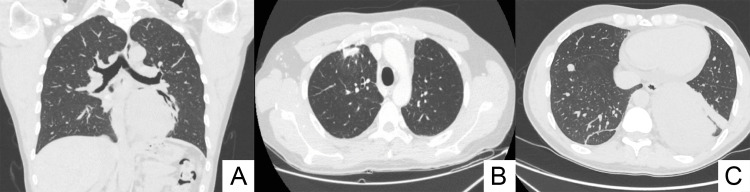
CT lung metastasis A) Lower pulmonary lobe completely occupied by mediobasal expansive formation,10x8cm, solid, inhomogeneous, extended in subpleural location, with signs of contiguity/compression in cranial location with the left atrium, at the confluence of the inferior pulmonary vein, the latter infiltrated and poorly recognizable in the proximal-medial section. B, C) Two solid, noncalcific nodular lesions of a secondary nature are appreciable in the anterior segment of the right upper lobe and antero-basal segment of the ipsilateral lower lobe, with dimensions of approximately 15 mm subpleural and 11 mm in the periscissural location.
